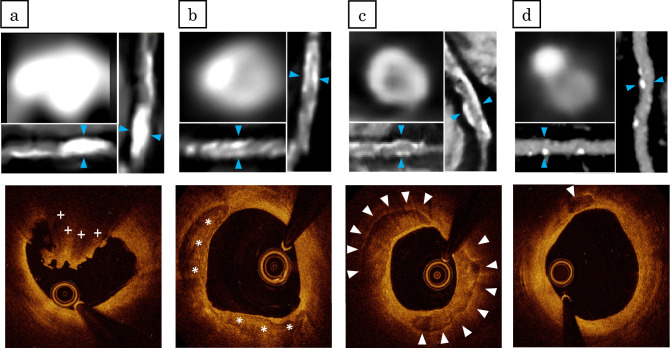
Representative CCTA and OCT images of calcified plaques. (a) A representative image of calcified nodule. CCTA image showing a large area of calcification. The area of calcified plaque on the cross-sectional image (at blue arrow heads) is 21.77 mm^2^. The OCT image of this lesion demonstrates calcified nodules (plus symbols). (b) A representative image of eccentric calcified plaque. The area of calcified plaque on the cross-sectional image (at blue arrow heads) is 2.8 mm^2^. The OCT image of this lesion demonstrates a superficial calcific sheet (asterisks). (c) A representative image of circumferential calcified plaque. CCTA image showing a circumferential calcification. The aread of calcified plaque on the cross-sectional image (at blue arrow heads) is 3.8 mm^2^. The OCT image of this lesion demonstrates a circumferential calcification (white arrow heads). (d) A representative image of spotty calcification. CCTA image showing a spotty calcification. The area of calcified plaque on the cross-sectional image (at blue arrow heads) is 0.8 mm^2^. The OCT image of this lesion demonstrated superficial small calcification (white arrow head). *CCTA* coronary computed tomography angiography, *OCT* optimal coherence tomography.
